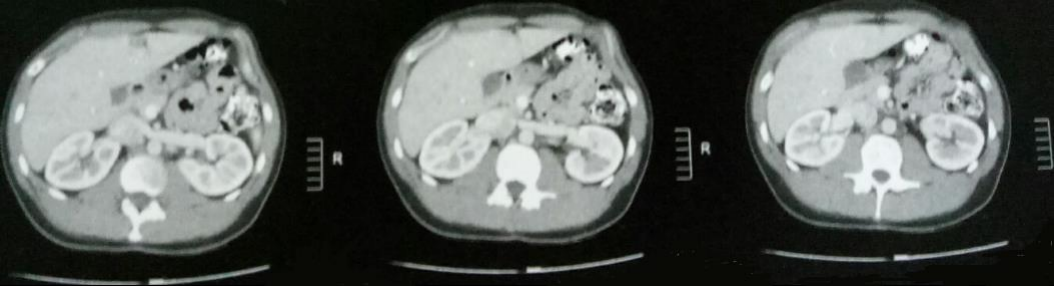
absence of renal masses on the abdominal CT scan
